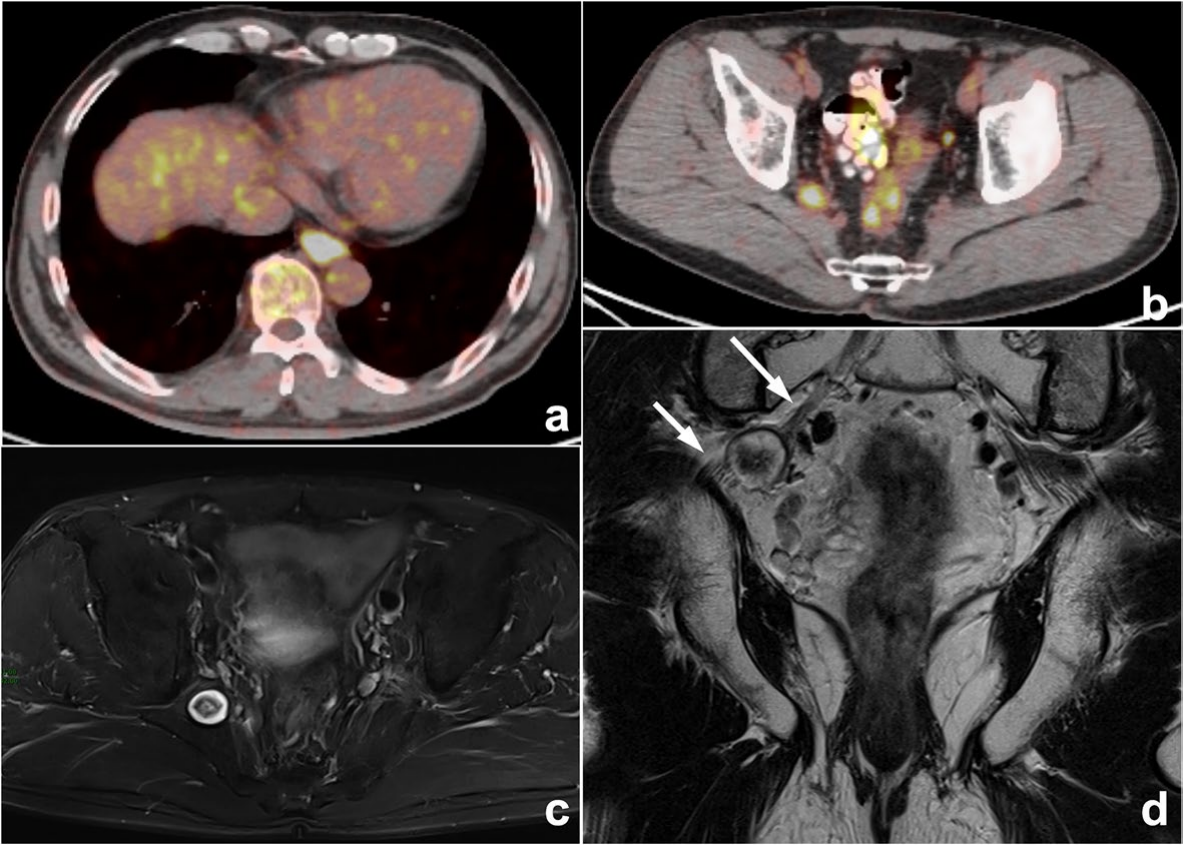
Esophageal carcinoma with multiple systemic metastases reported by PET-CT in a 60-year-old man with dysphagia for 1 month. a, b PET-CT images showed a mildly elevated pelvic uptake nodule in the right pelvis, which was suspected to be metastasis. c, d Therefore, the radiologist was influenced by the previous esophageal carcinoma and PET-CT reports, ignoring typical neurogenic tumor signs such as the “target sign” and the close association with the sacral plexus nerve (arrows). Eventually, the right pelvic nodule was misdiagnosed as metastasis in the MRI report. This is a typical example of prior report bias
